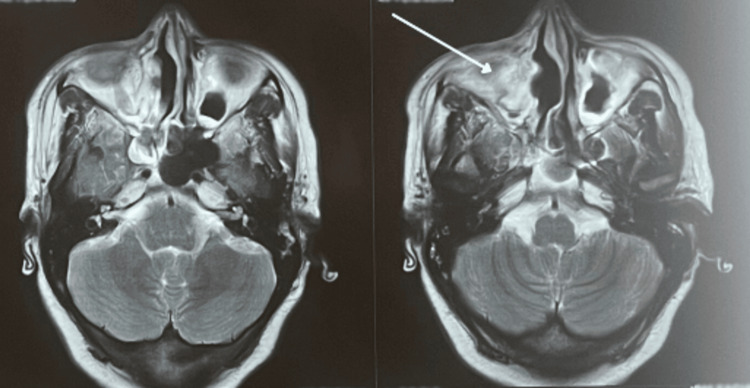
Facial MRI revealed a tumoral process centered on the right maxillary sinus, with locoregional infiltration, in relation to a residual focus.
